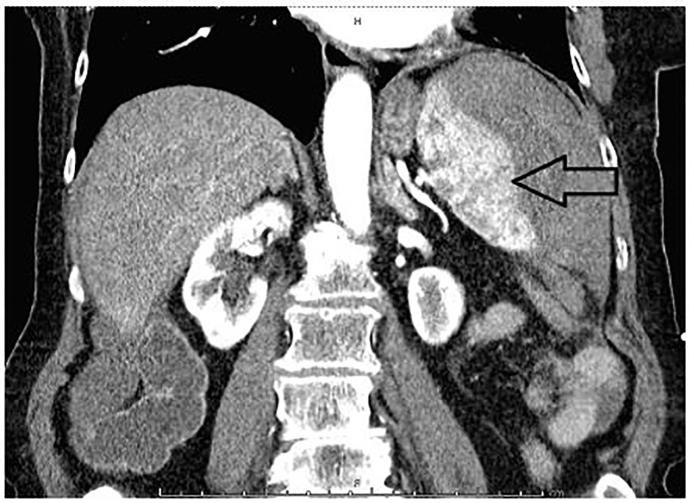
Computed tomography of abdomen and pelvis in coronal view showing splenic laceration.
